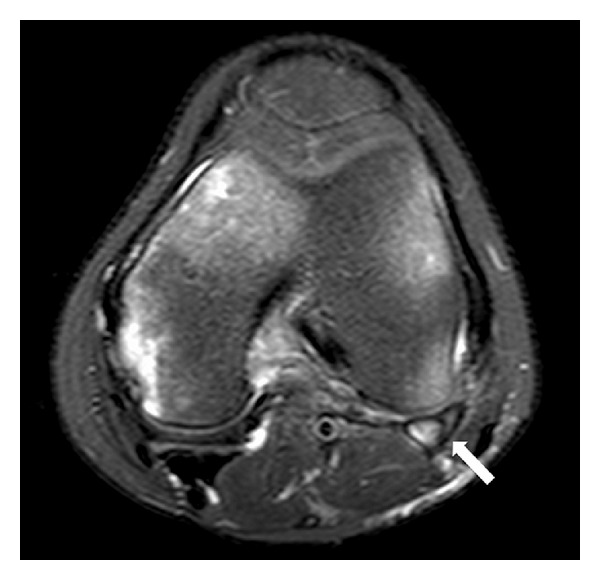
Axial T2-weighted fat-suppressed MRI depicted a low signal line within the fabella consistent with fracture (white arrow).
